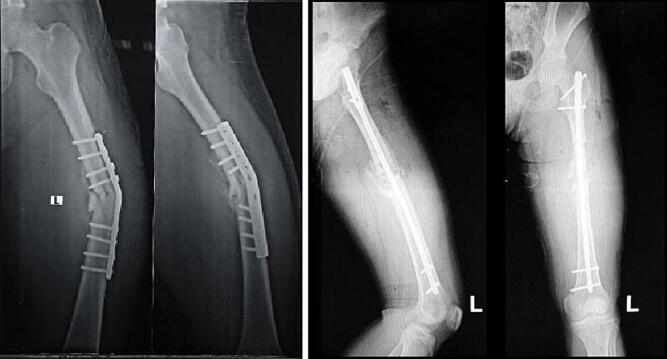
X-ray 2 months after the first surgery showed implant failure. It was then revised with IM nailing.
